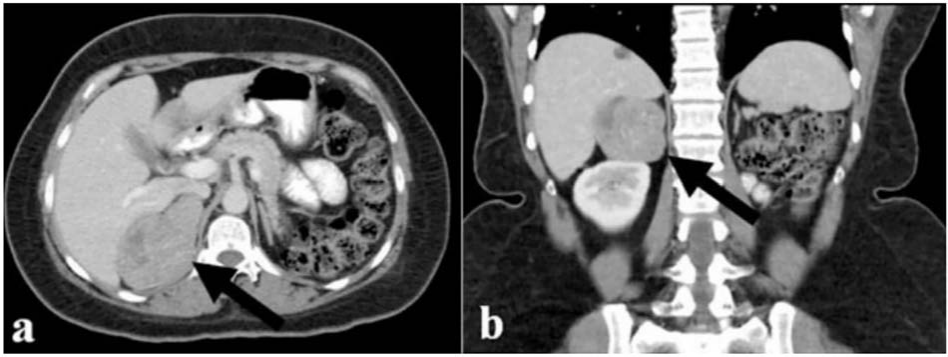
(a) and (b) The abdominal CT scan showed a large right adrenal tumor.
